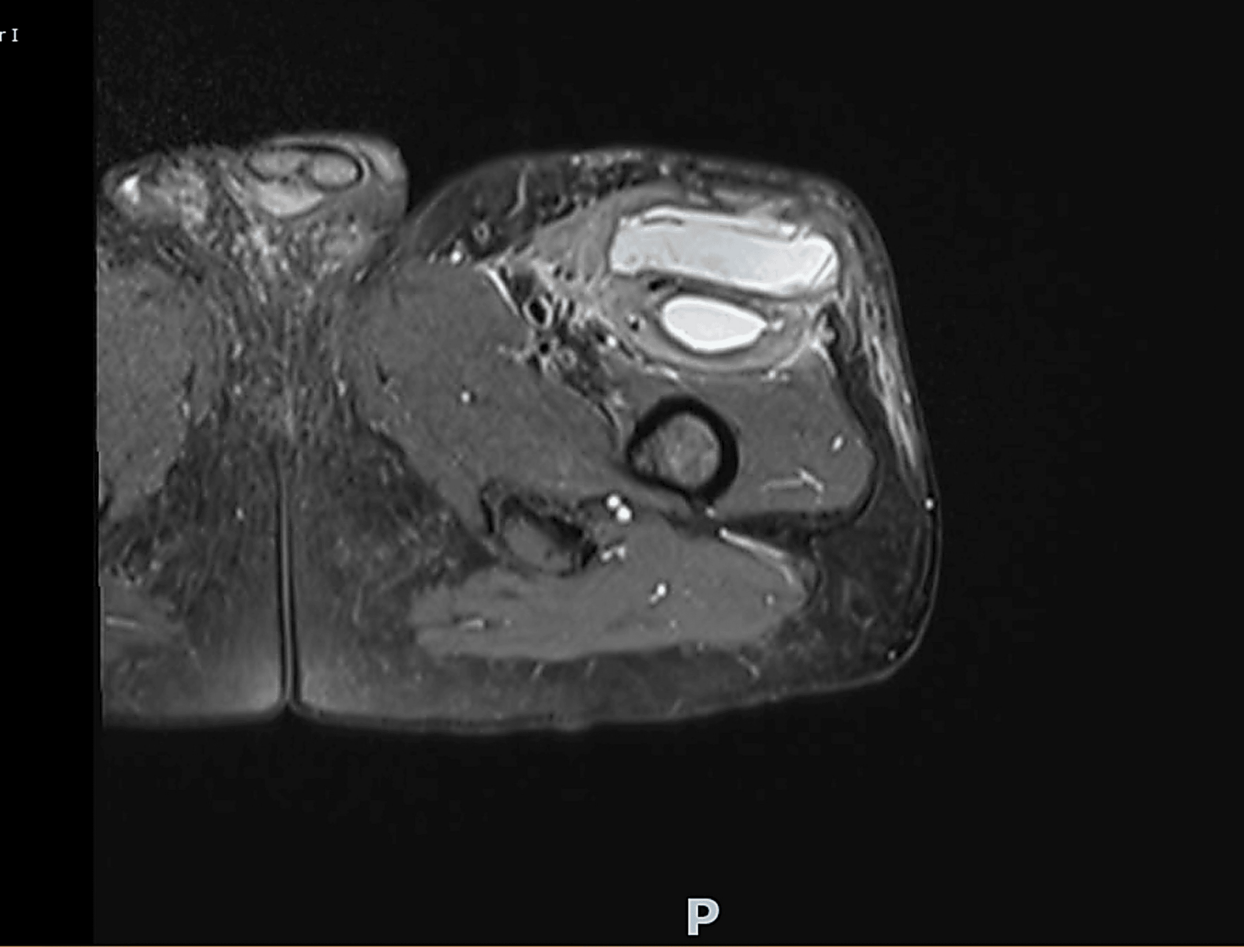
MRI of the left thigh revealing a large multiloculated collection (~15 × 6.5 × 4 cm) with associated myositis in the left thigh muscles, without evidence of soft tissue neoplasm
